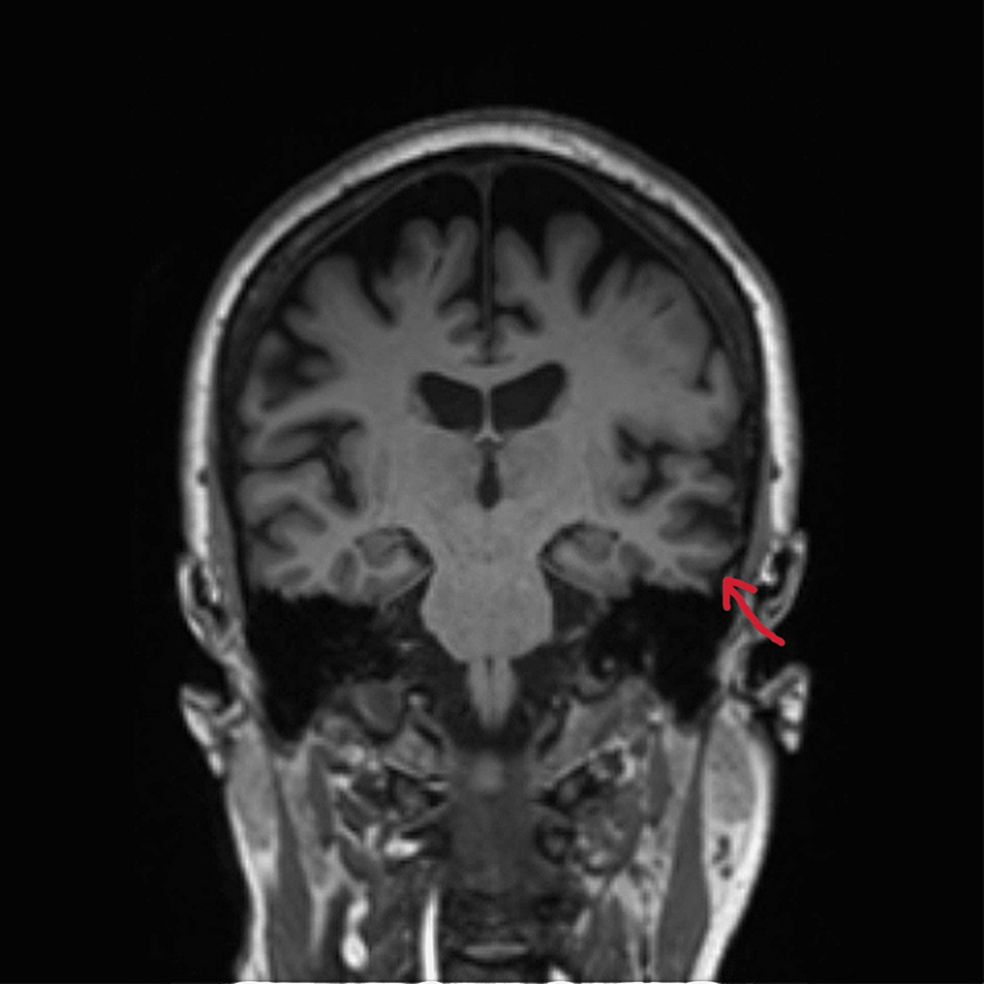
MRI Brain Coronal T1 indicating generalised cortical atrophy with prominent atrophy in parietal and occipital regions
